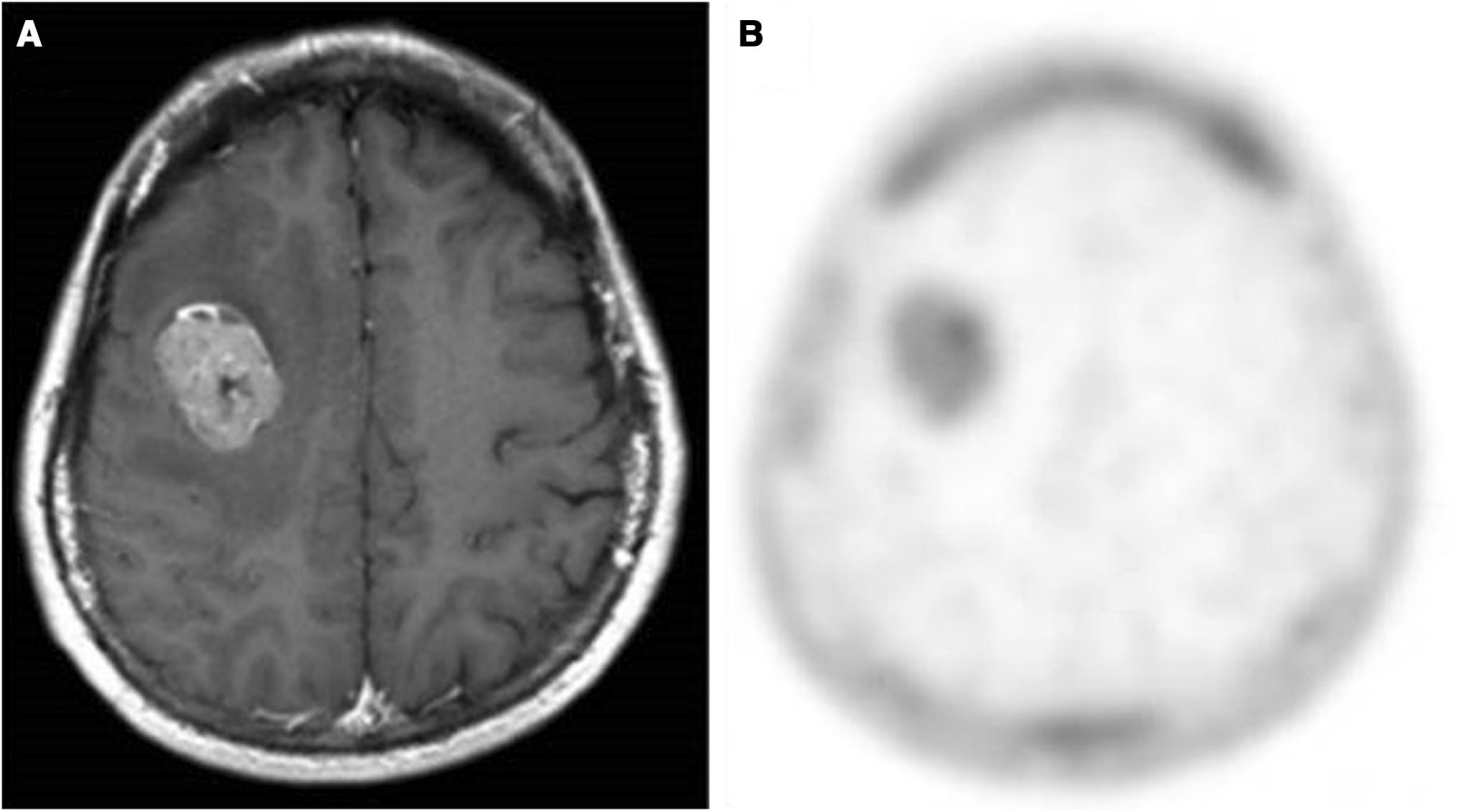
Newly diagnosed grade IV glioblastoma. a Magnetic resonance image with contrast enhancement, b
